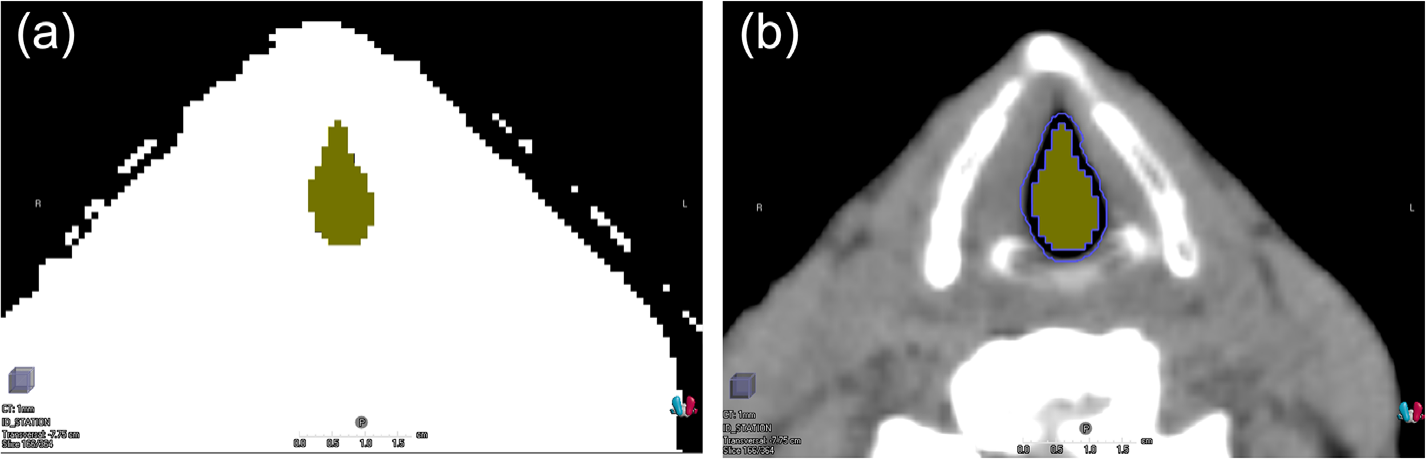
Axial CT images of the ROI delineation for material override in the glottic air cavity and surface displayed using two window level/width settings: (a) window level/width determined for the air–tissue boundary (–500 HU/0 HU); (b) soft tissue window level/width (40 HU/350 HU). The yellow wash represents the air ROIs of the glottic air cavity, and the blue contour represents the water ROIs of the glottic surface. CT, computed tomography; HU, Hounsfield unit; ROI, region of interest.
